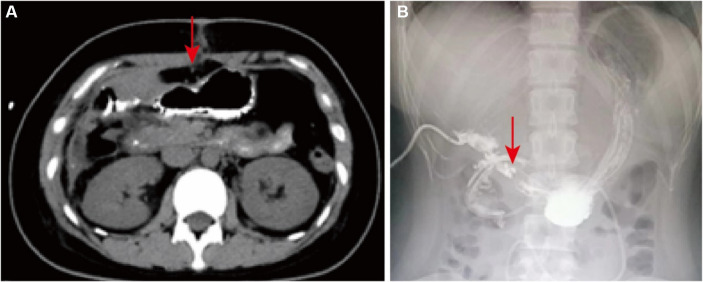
Diagnostic results. (A) The abdominal CT image. The red arrow points to pneumoperitoneum. (B) The upper gastrointestinal imaging finding. The red arrow points to the fistula.
